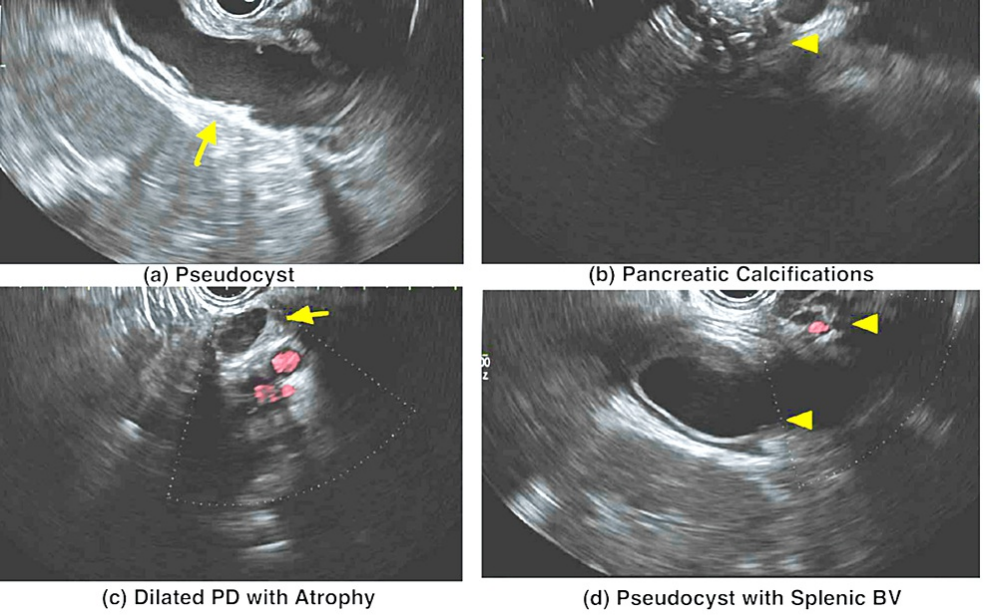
EUS demonstrating (a) a pseudocyst (yellow arrow), (b) pancreatic calcifications (arrowhead), (c) dilated PD with atrophy, and (d) the splenic blood vessels close to the pseudocyst. EUS: endoscopic ultrasound; PD: pancreatic duct; BV: blood vessels
